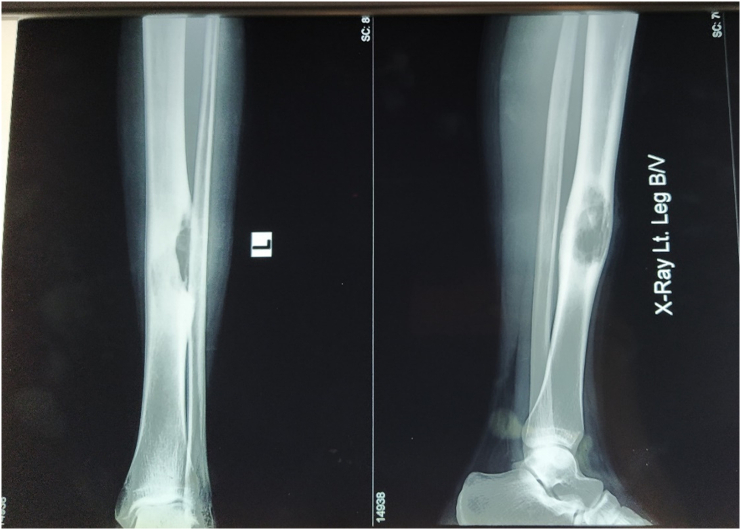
X-ray of left leg showing osteolytic, expansile, eccentric lesion with sclerotic bone margin on the diaphysis of tibia.
